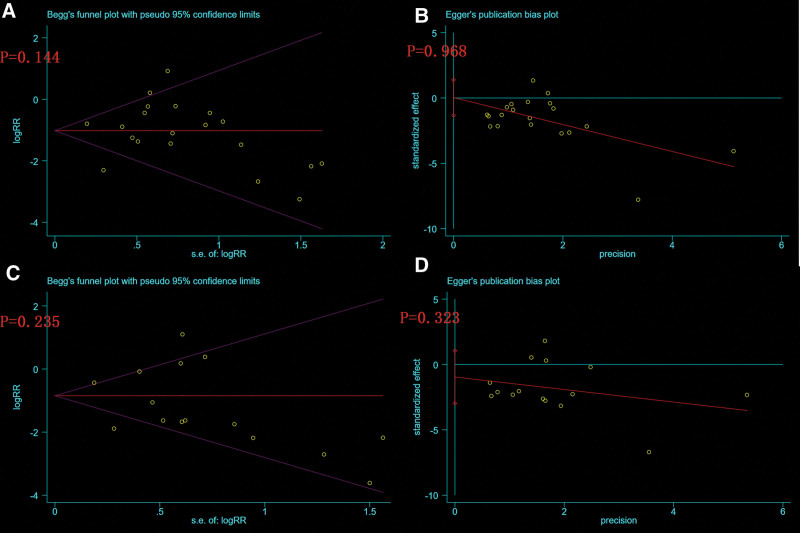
Publication bias plot of Begg’s test and Egger’s test of THA conversion rate and radiographic progression rate. (A) Begg’s test of THA conversion rate (*P* = .144); (B) Egger’s test of THA conversion rate (*P* = .968); (C) Begg’s test of radiographic progression rate (*P* = .235); and (D) Egger’s test of radiographic progression rate (*P* = .323). THA = total hip arthroplasty.
